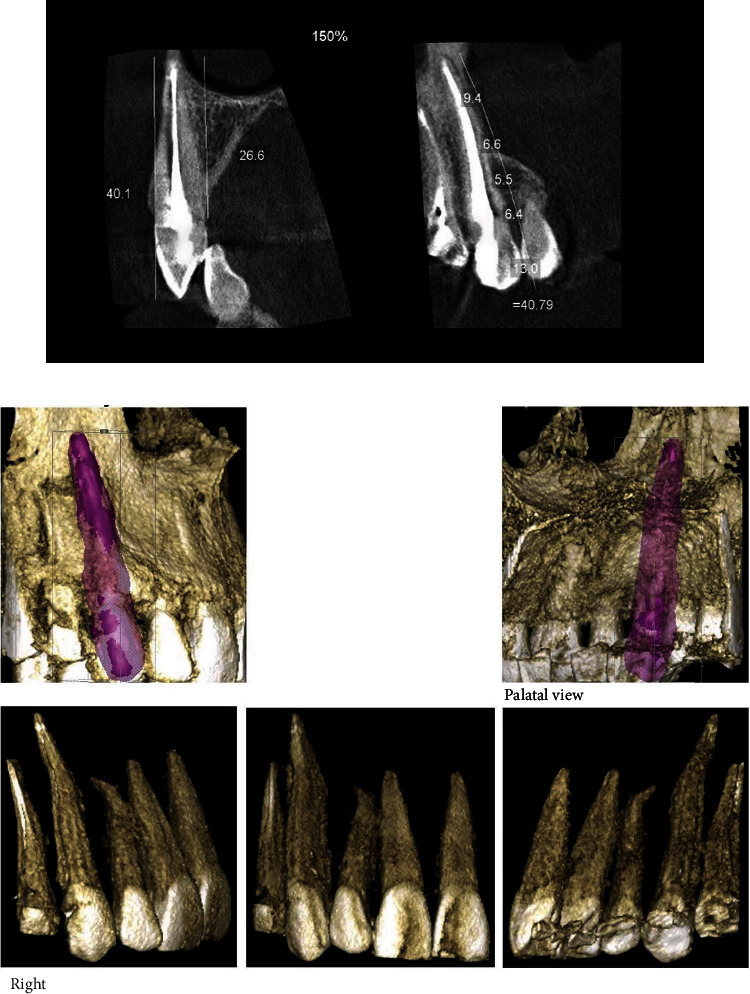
Posttreatment CBCT radiographs in six-month follow-up. (a) Coronal (left) and sagittal (right) slices. (b) 3D model of the treated tooth.
